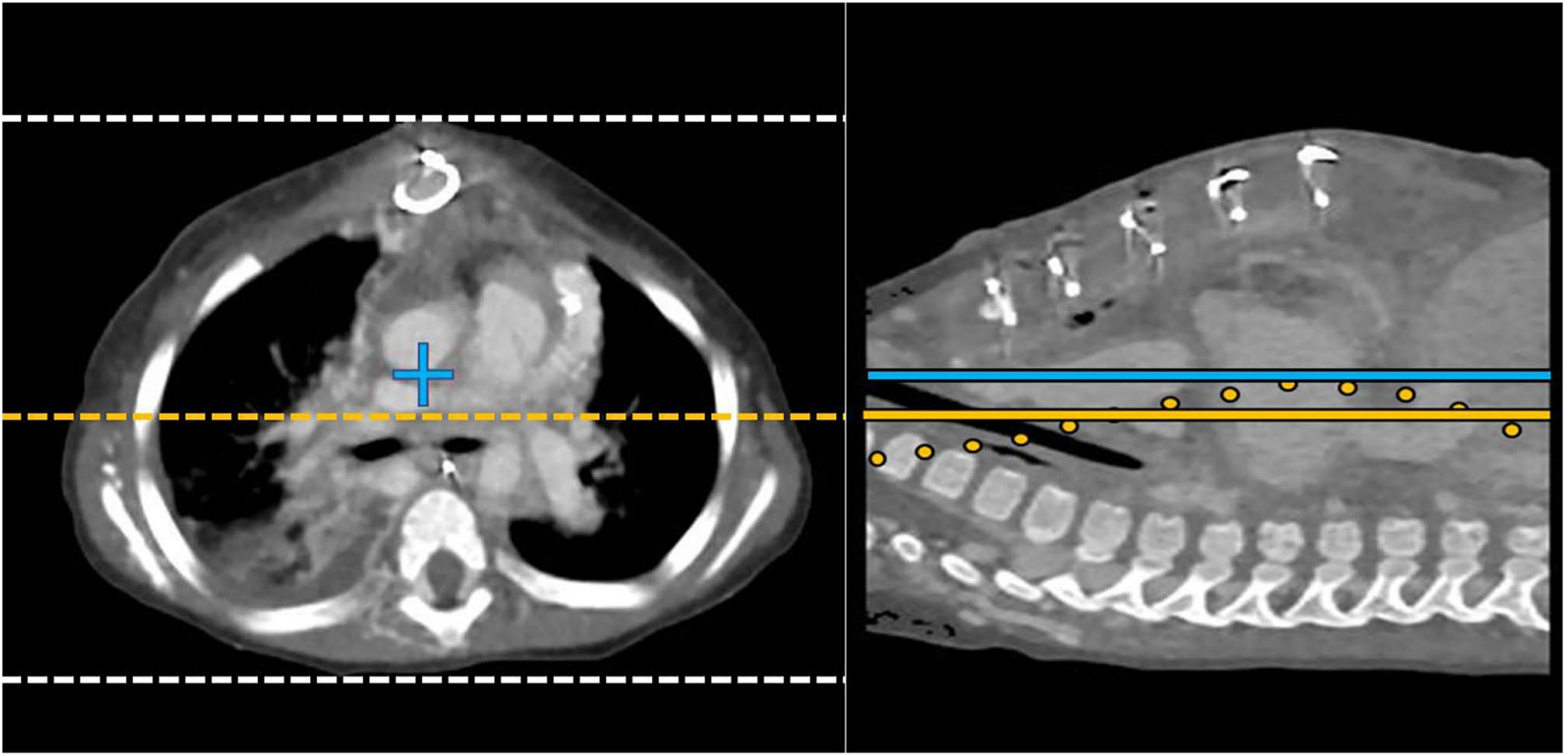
Definition of patient isocenter. (a) Axial image binarized with the CT value threshold to extract the patient isocenter, defined as the middle point (yellow dotted line) between the highest and lowest point (white dotted lines) of the extracted skin surface. All images were reconstructed so that the center of the image coincides with the isocenter of the gantry (blue cross). (b) Sagittal image showing the grand truth vertical center of patients, calculated using the averaged center values of each image along the scan length (yellow line). The patient was positioned below the isocenter of the gantry (blue line).
